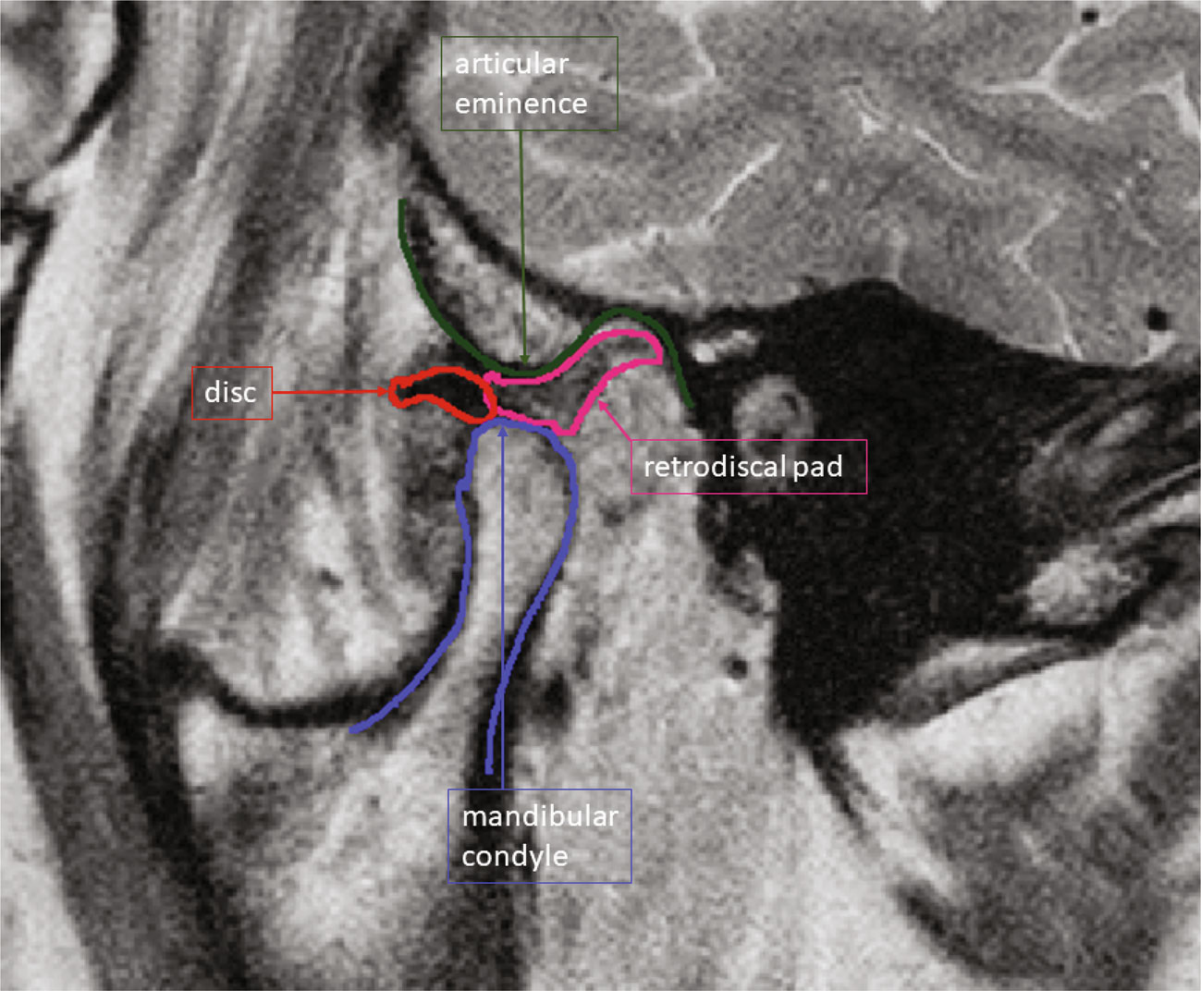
Dynamic MRI of the TMJ at 10-week follow-up with patient's mouth in the open position revealed persistent left disk displacement, however, without signs of inflammation or effusion. The condyle moved further forward than in the first MRI examination and reached the top of the articular eminence.
